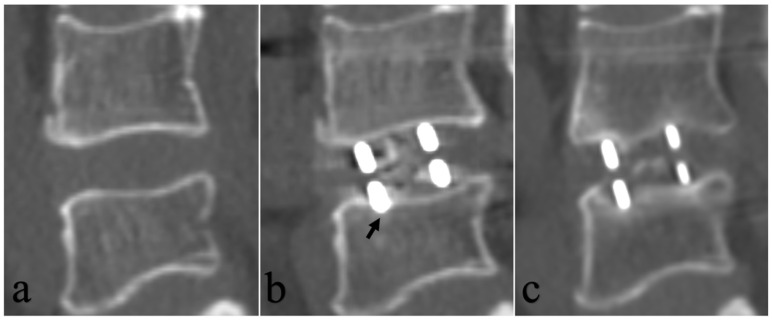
Case 1. Images obtained in a 56-year-old man with BMD (95.0 mg/cm^3^) and presence of intraoperative endplate injury following OLIF with bilateral posterior pedicle screw fixation. (a) Preoperative CT sagittal reconstruction. (b) CT sagittal reconstruction at 3 days postoperatively showing intraoperative endplate injury (the area of endplate injury indicated by arrow). (c) CT sagittal reconstruction at 3.3 years postoperatively showed the presence of CS.
